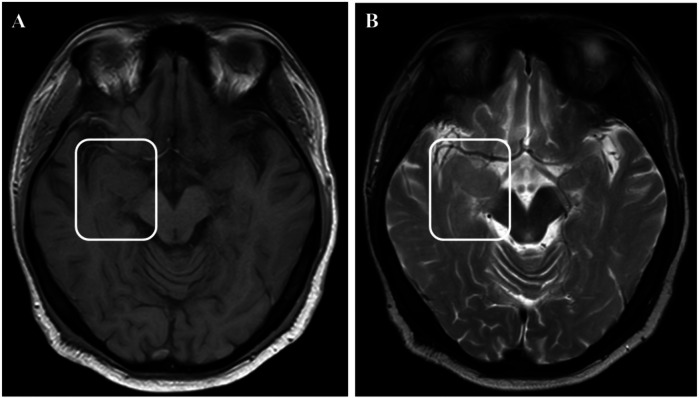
MRI images showed enlargement of the right amygdala and hippocampi. (A) showed T1 weight image. (B) showed T2 weight image.
